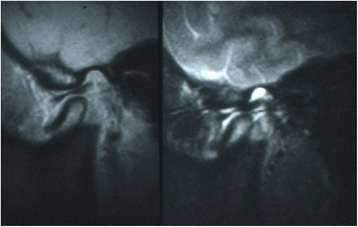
On proton density-weighted images in the closed-mouth position, the condylar head and disc show a downward displacement with normal condyle-disc relation and disc shape. On T2-weighted image in the closed-mouth position, high signal intensity is found in posterosuperior joint space. It is regarded as a hemarthrosis
